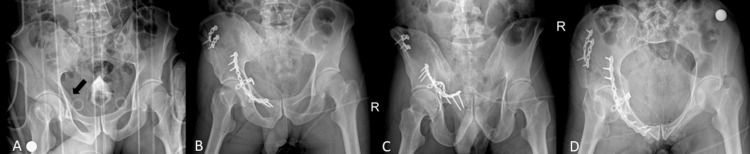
Preoperative and postoperative radiographs A) preoperative pelvis anterior-posterior (AP) radiograph, B) postoperative pelvis AP radiograph, C) postoperative pelvis outlet radiograph, D) postoperative pelvis inlet radiograph
